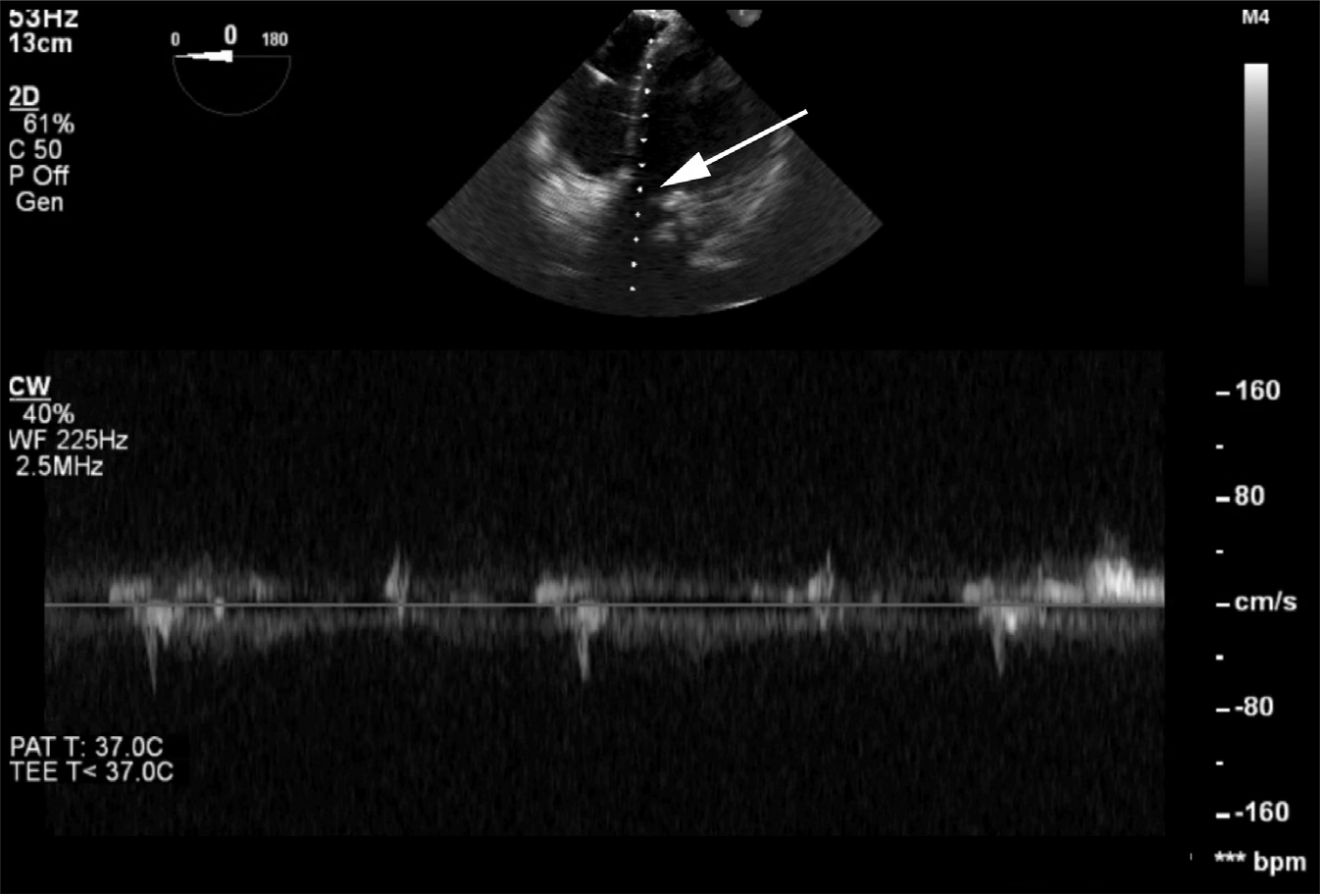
Doppler Interrogation of the Aortic Valve The image (the arrow denotes the line of interrogation passing through the left ventricular outflow tract) indicated no flow through the prosthesis.
